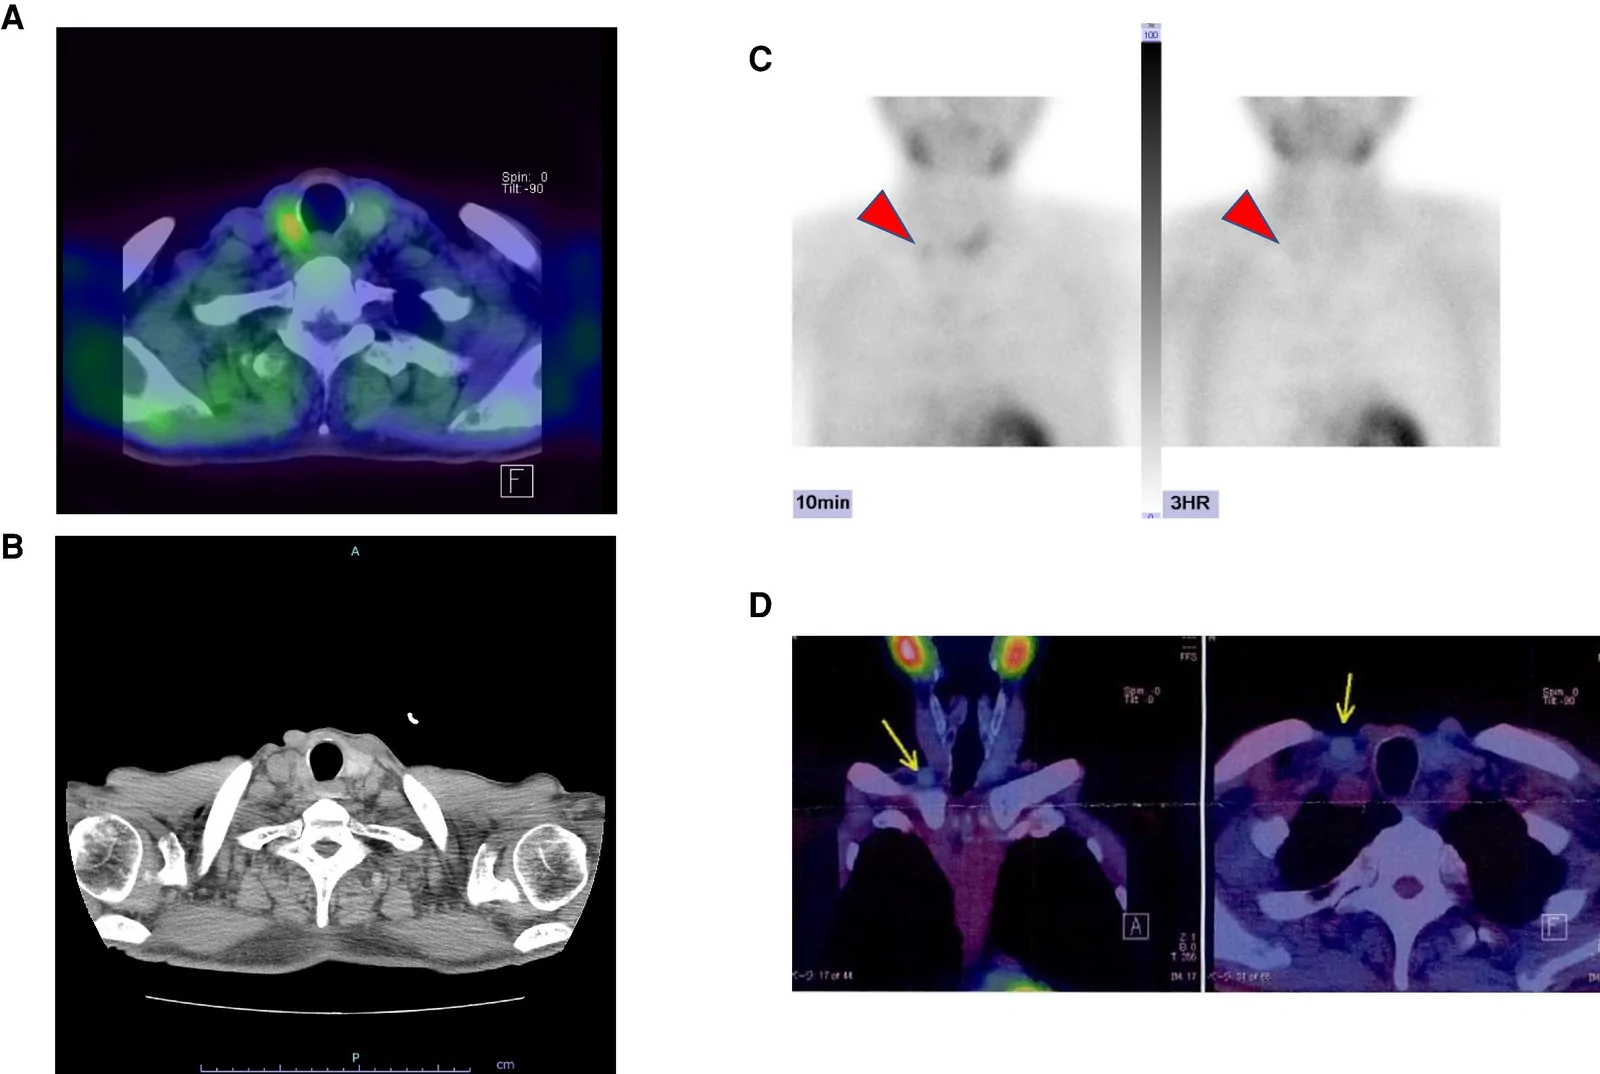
Imaging findings during the workups for the third and fourth surgical procedures (Px3 and Px4). A and B) A coronal fused single-photon emission computed tomography/computed tomography (SPECT/CT) image (A) and a CT scan (B) obtained before Px3, 15 years after the initial diagnosis of hypercalcemia. Arrows indicate the site of focal 99mTc-MIBI accumulation in (A) and the corresponding anatomical lesion on CT in (B). C and D) Imaging findings obtained during the workup for Px4, 18 years after the initial diagnosis, include a 99mTc-MIBI scan (C) showing increased tracer uptake in the right supraclavicular region (arrows) and a coronal fused SPECT/CT image (D) showing faint abnormal 99mTc-MIBI uptake in the right supraclavicular region (arrow).
